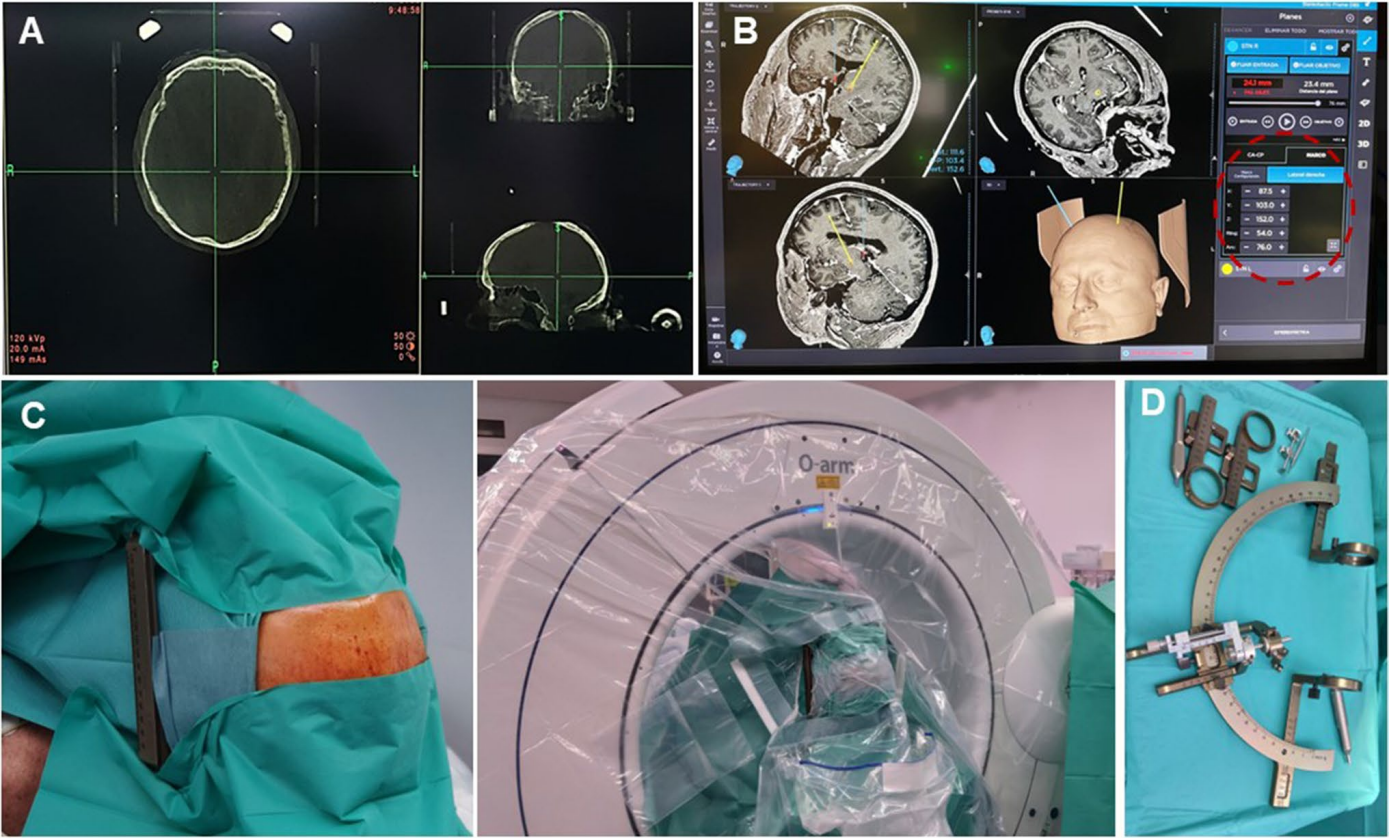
Intraoperative programming and preparation. A The intraoperative CT 3D image is fused with preoperative planning, and B coordinates are changed from AC-PC reference to frame-based reference. Adjustments are needed to entire figures or 0.5 decimals. C The field is draped, leaving the laterals of the frame uncovered. D The coordinates are transferred to the stereotactic frame
